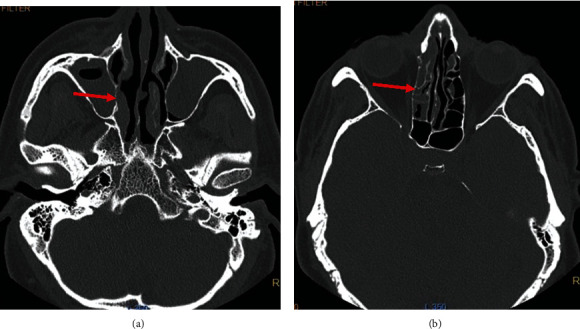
Axial paranasal CT images without contrast administration showing (a) mucosal thickening and secretion in the right maxillary sinus and a retention cyst in the Lt maxillary sinus and (b) mucosal thickening and secretion in the right ethmoid sinus with a lesser degree in the Lt ethmoid sinus.
